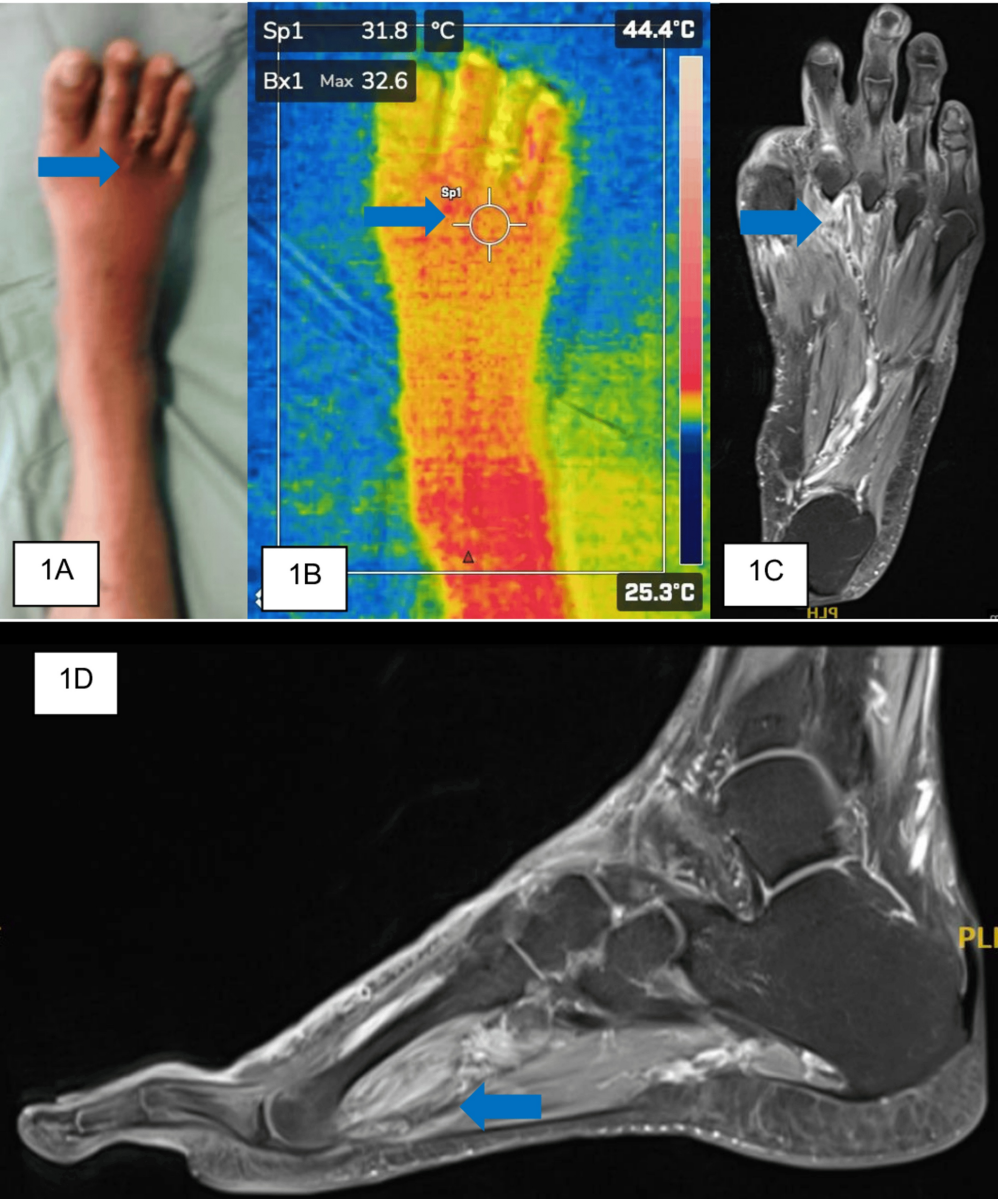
(A) Clinical photo of the affected foot with the area of interest marked by the blue arrow. (B) Thermal imaging of the affected foot upon admission to the ward. (C) Axial view of the T1 post-contrast MRI image with the affected area (arrow). (D) Sagittal view of the T1 post-contrast MRI image clearly delineating the area of interest (blue arrow).
